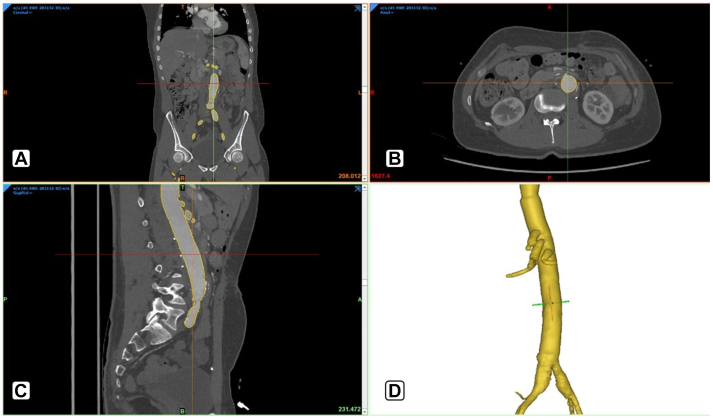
Postoperative computed tomography angiography (CTA) data set of the patient: (A) coronal view; (B) axial view; (C) sagittal view; and (D) three-dimensional (3D) segmented reconstruction.
